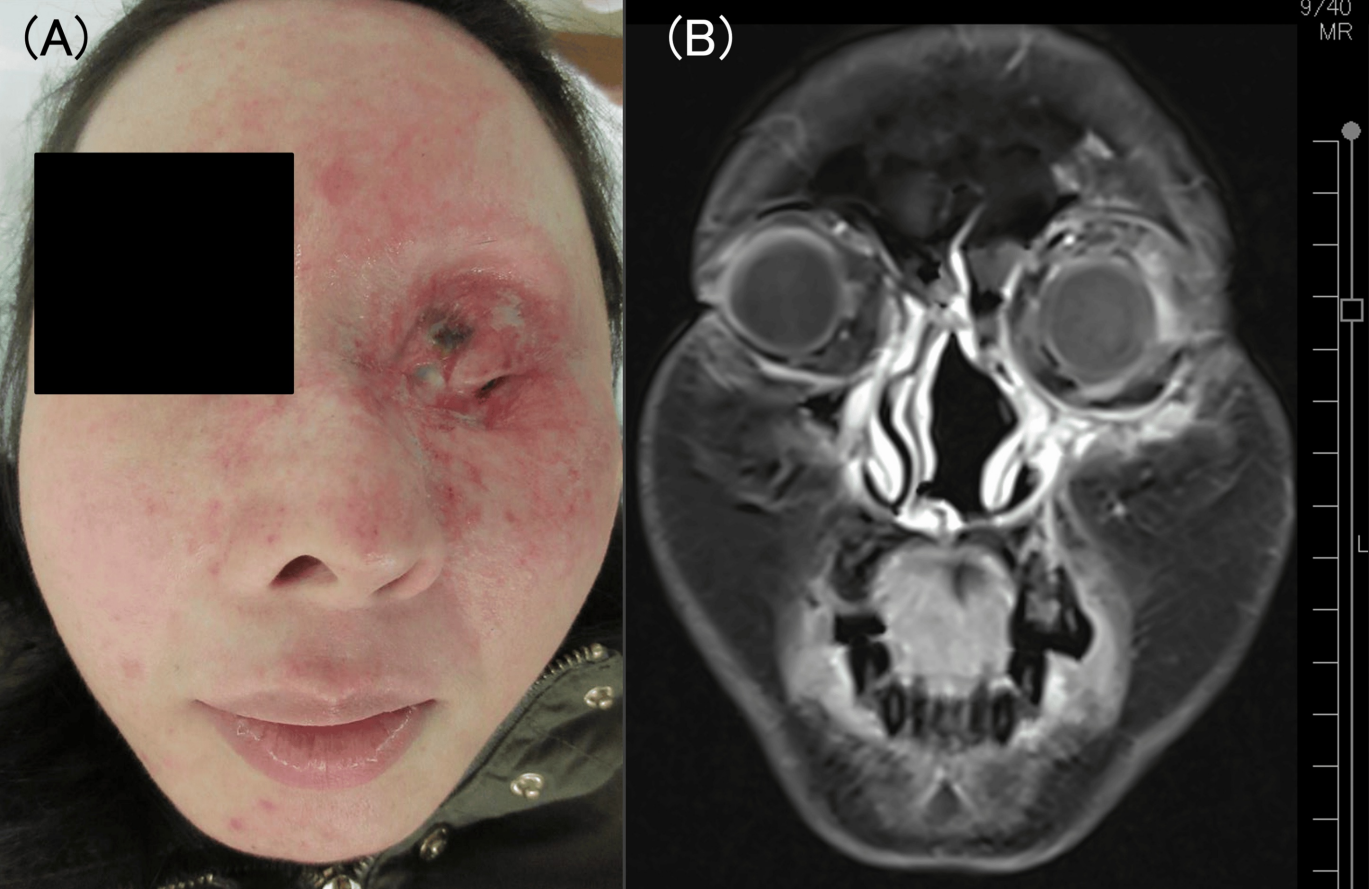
Patient’s face and MRI image 24 months after treatment. (A) The eyelid tumor completely disappeared; however, the patient lost eyesight in the left eye. (B) The coronal T1-weighted contrast-enhanced MRI showing complete remission MRI: magnetic resonance imaging
